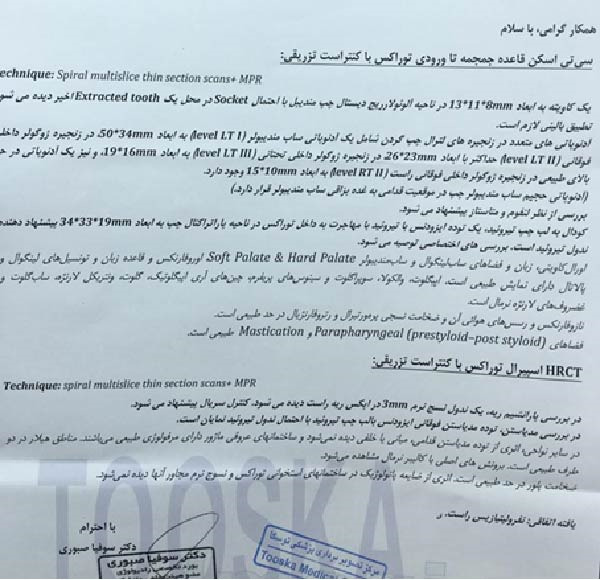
Contrast‐enhanced CT scan from the skull base to the thoracic inlet.
